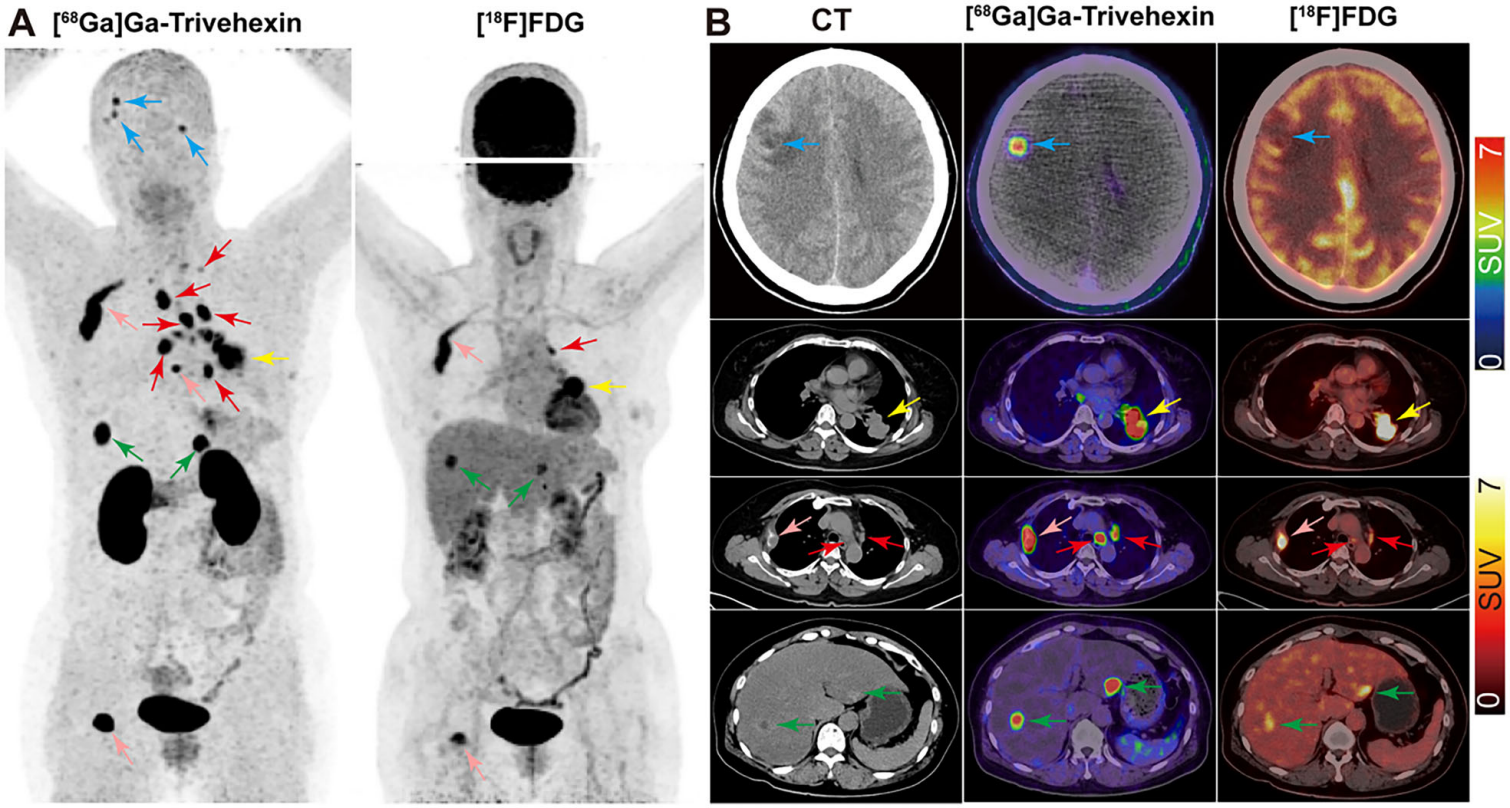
Representative [^68^Ga]Ga‐Trivehexin PET/CT images of a 55‐year‐old woman with lung adenocarcinoma and multiple metastatic lesions (cT2bN3M1c, IVB) compared with [^18^F]FDG PET/CT. The maximum intensity projection (MIP; A), axial CT, and fused images (B) of [^68^Ga]Ga‐Trivehexin and [^18^F]FDG PET/CT showed the primary tumor (SUVmax, 20.66 vs 12.45; yellow arrows) and metastatic lesions including lymph nodes (SUVmax, 23.88 vs 7.44; red arrows), brain (SUVmax, 3.39– 6.85 vs 9.78–12.89; TBR_b_, 96.23–195.66 vs 1.05–1.39; blue arrows), liver (SUVmax, 22.72 vs 7.05; green arrows) and bone metastases (SUVmax, 20.38 vs 11.19; pink arrows). TBR_b_, tumor‐to‐background (normal brain parenchyma) ratio.
